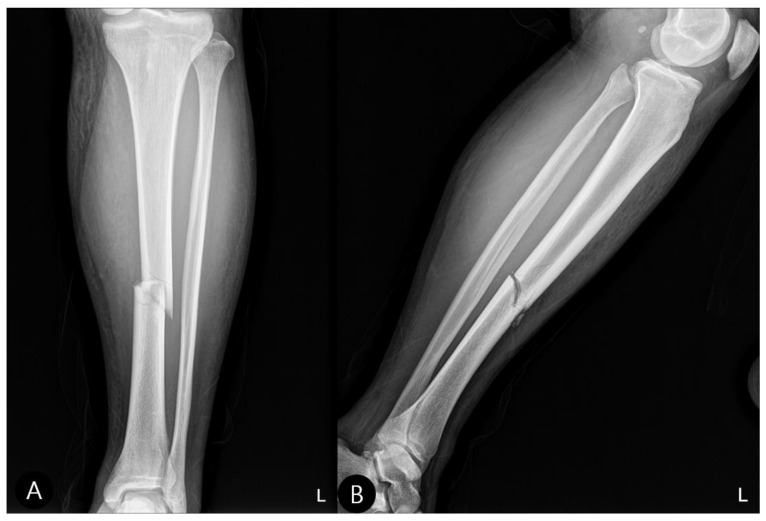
Initial tibia radiographs (2 October 2023). (A) Anteroposterior (AP) view showing a displaced fracture of the tibial shaft. (B) Lateral view confirming a mid-shaft tibia fracture. “L” indicates the left side.
